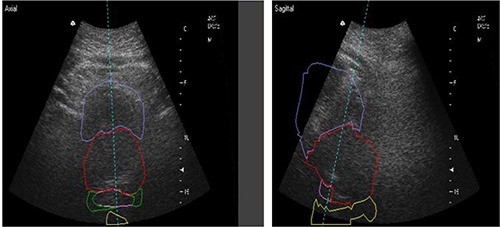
Axial and sagittal ultrasound images shown superimposed with bladder, prostate, seminal vesicles, and rectum contours from the treatment planning CT.
